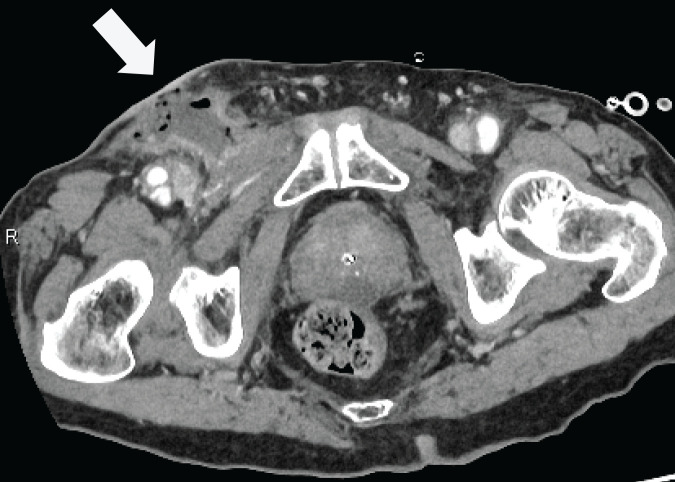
Preoperative contrast-enhanced CT imaging showing a small bowel hernia in the right groin. The intestinal wall within the hernia sac appeared indistinct, accompanied by increased subcutaneous fat tissue density and the formation of a skin fistula (arrow).
